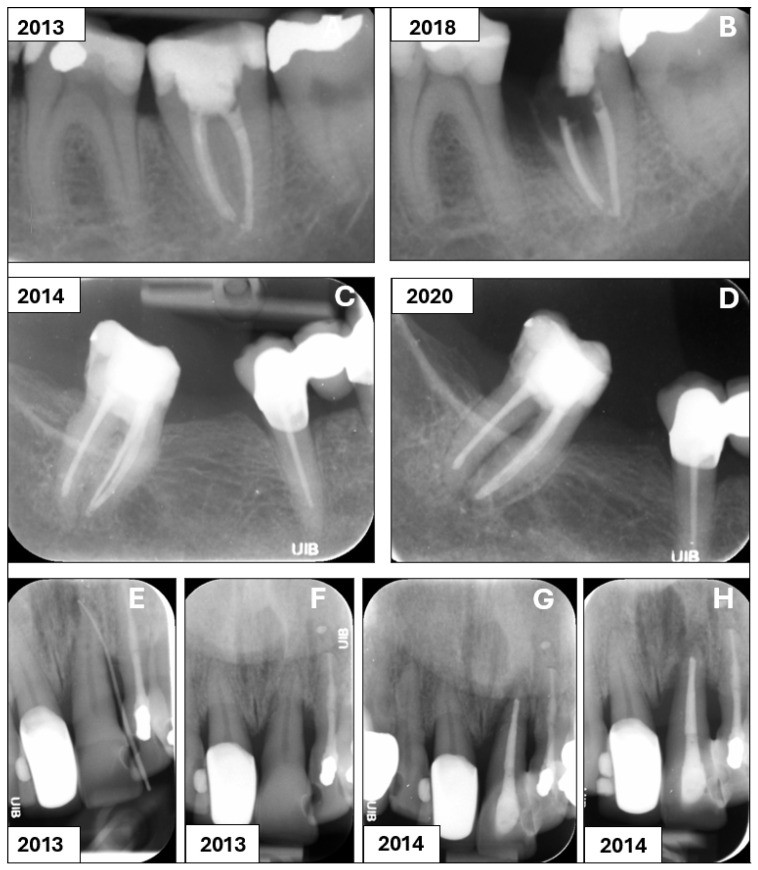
Representative radiographs of teeth extracted due to carious lesion (A,B), tooth fracture (C,D) and periodontal disease (E–H). Tooth 37 was root filled in 2013 (A). An expanding carious lesion resulted in an unrestorable tooth that eventually was extracted in 2018 (B). Tooth 47 was root filled in 2014 (C). In 2020, the patient experienced severe pain of sudden onset for 2 days. Clinical examination showed endodontic pocket to apex and tooth was extracted due to fracture (D). Tooth 21 was diagnosed with pulp necrosis and periapical abscess with sinus tract (E,F). Tooth was root filled in 2013, and a six-month (G) and one-year recall (H) showed progression of periodontal disease. Tooth 21 was eventually extracted.
